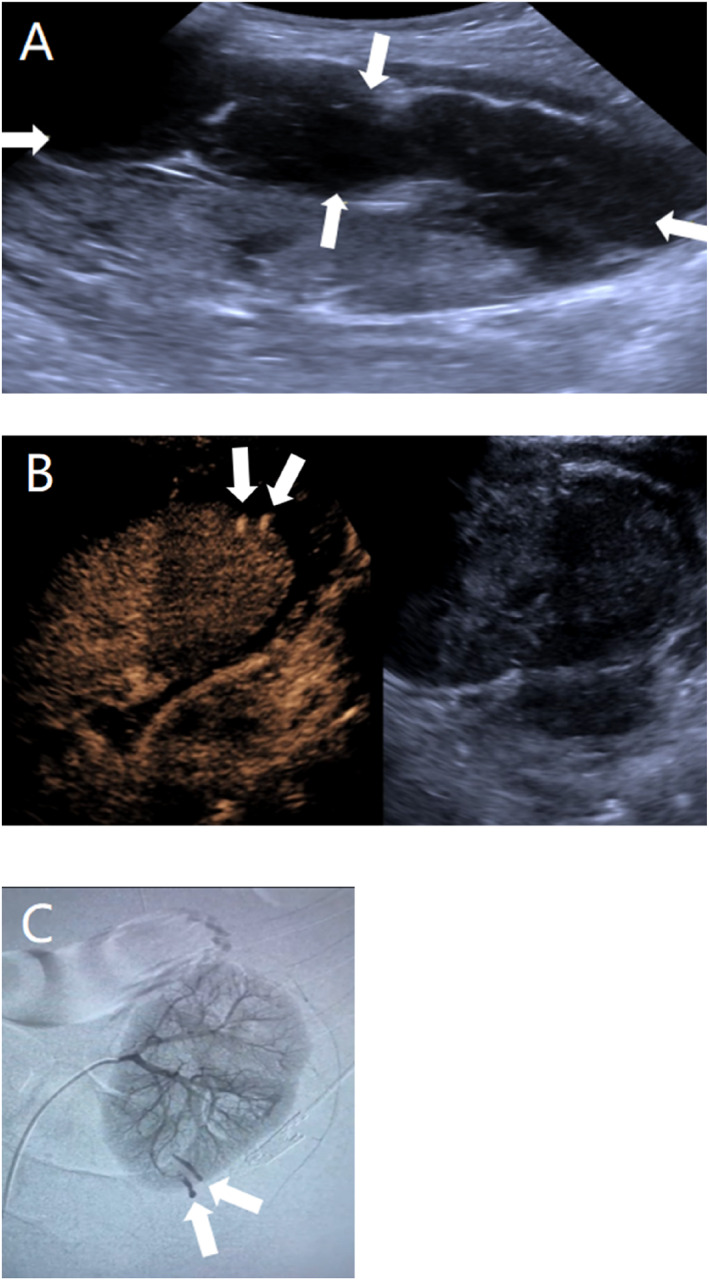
An 8.5‐year‐old female, clinically diagnosed with antineutrophil cytoplasmic antibody (ANCA)‐associated nephritis, 1 day after left renal puncture. (A) Conventional ultrasound image showing hypoechoic and echoless areas under the perinephric peritoneum of the left kidney. (B) Two ultrasound contrast leaks from the left posterior inferior renal branch artery (arrows). (C) Intraoperative DSA image showing that the left renal artery was well visualized and that two contrast leaks could be observed in the posterior inferior branch artery of the left kidney (arrowheads).
